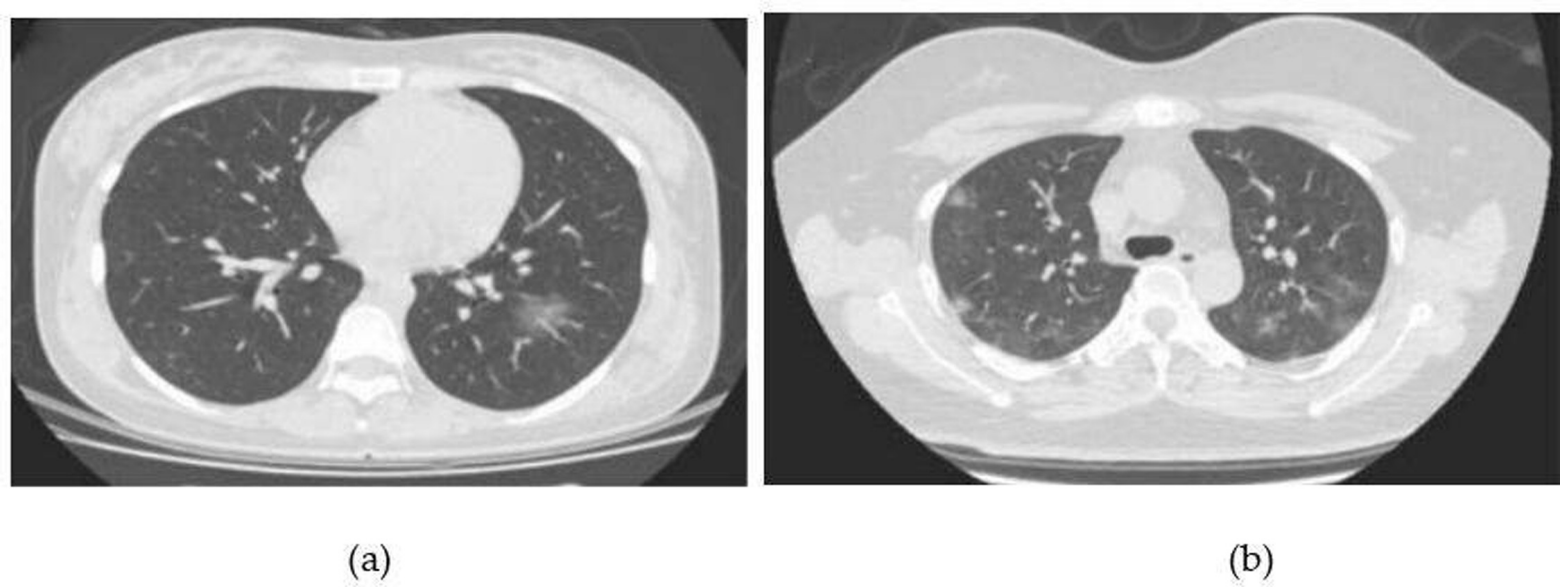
Typical CT images of patients: (a) clinical common patients; (b) the main manifestations of GGO shadows in patients.
